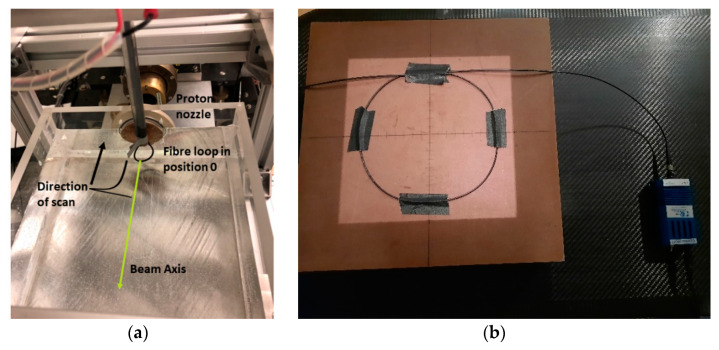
(a) setup of the fibre loop in the water box for proton radiation (b) setup of the fibre loop on a water equivalent material for X-ray radiation.
